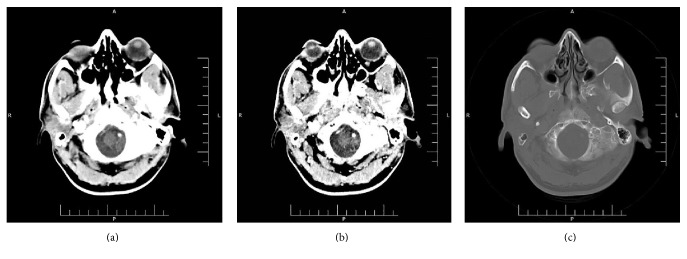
(a) Tri-dimensional CT scan reveals increasing of the thickness of right maxillary sinus soft tissue. (b) Massive destruction of right maxillary body. (c) Right maxillary sinus opacity with destruction of sinus wall.
